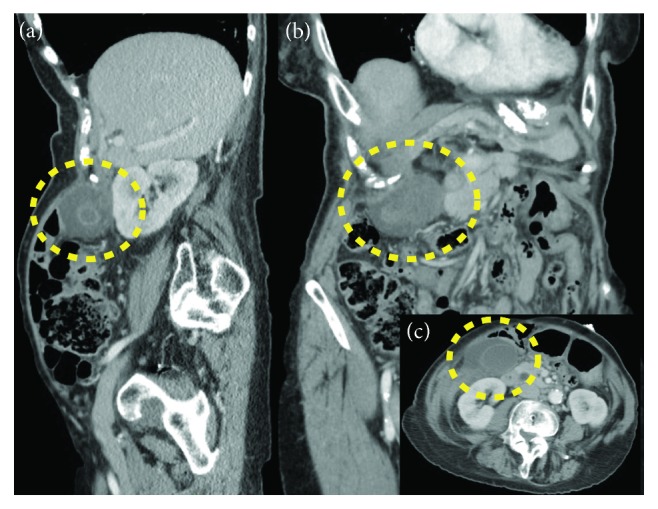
CT scan: (a) sagittal plane; (b) coronal plane; (c) axial plane. Dotted line circle shows the site of the gallbladder. Unique lithiasis is observed in (a) and (c).
